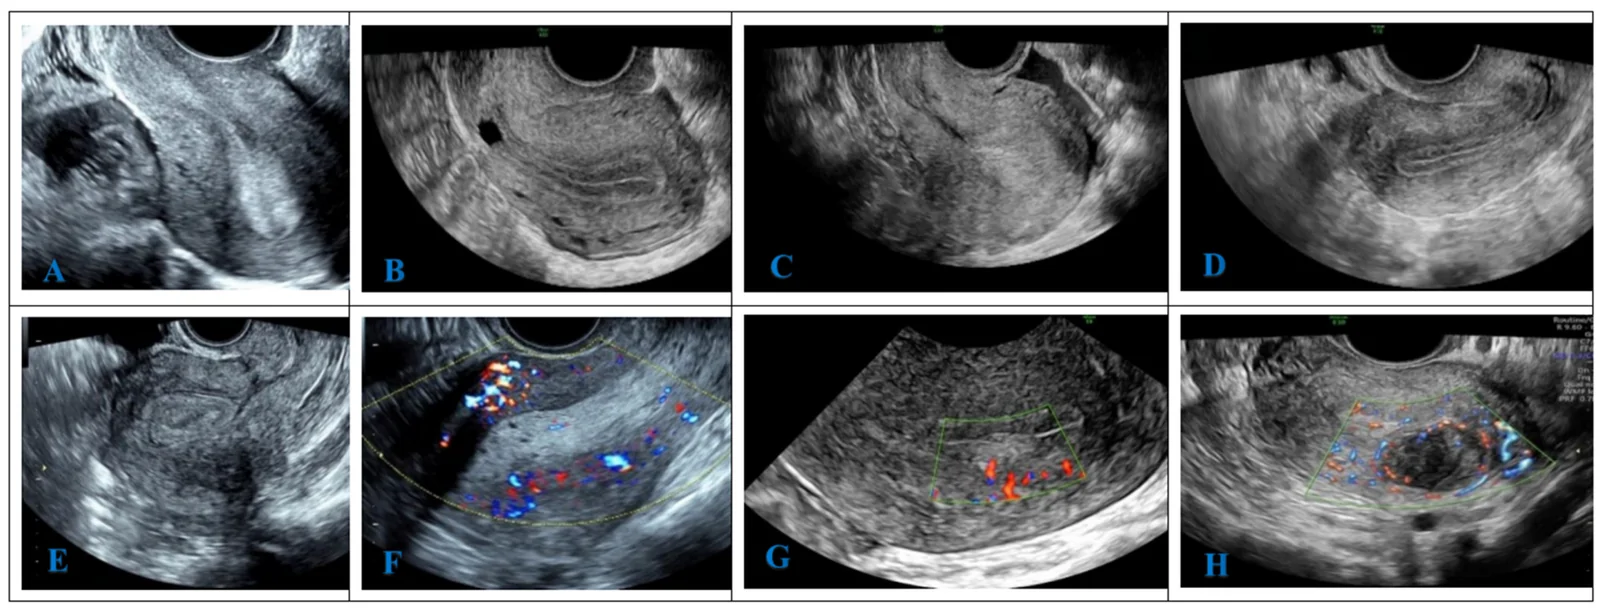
Images of benign IETA ultrasonographic features. (A) Homogeneous hyperechoic; (B) Three-layer pattern; (C) Homogeneous isoechoic; (D) Endometrial midline appearance: “linear” and endometrial–myometrial junction: “regular”; (E) The “bright edge” sign, the echo formed by the interface between an intracavitary lesion and the endometrium; (F) Homogeneous with regular cysts; (G) Vascular pattern: single vessel (without branching); (H) Vascular pattern: circular vessels.
